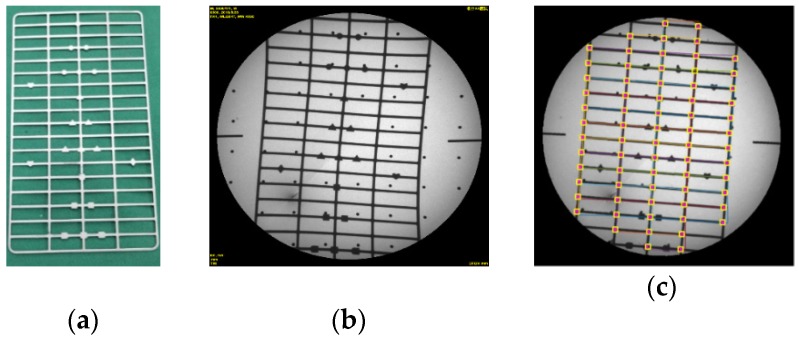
Image rectification test: (a) Test grid plate; (b) Raw X-Ray image of test plate; (c) Rectified Images. “×” represented the position of the grid points of the test plate in the raw image, “——” represented the grid distribution of the test plate in the raw image and □ represented the ideal position of the test plate.
